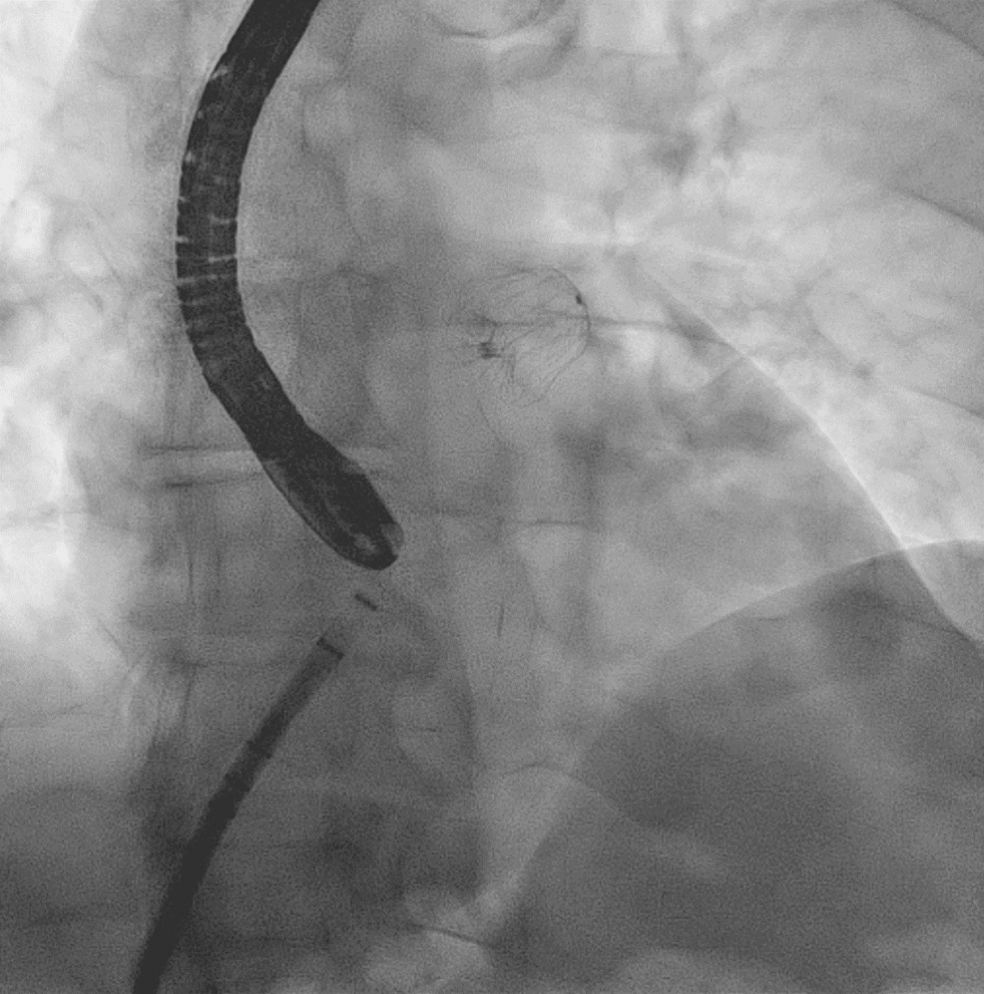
The deployed left atrial appendage occluder Echocardiographic monitoring confirmed the correct positioning of the device.
